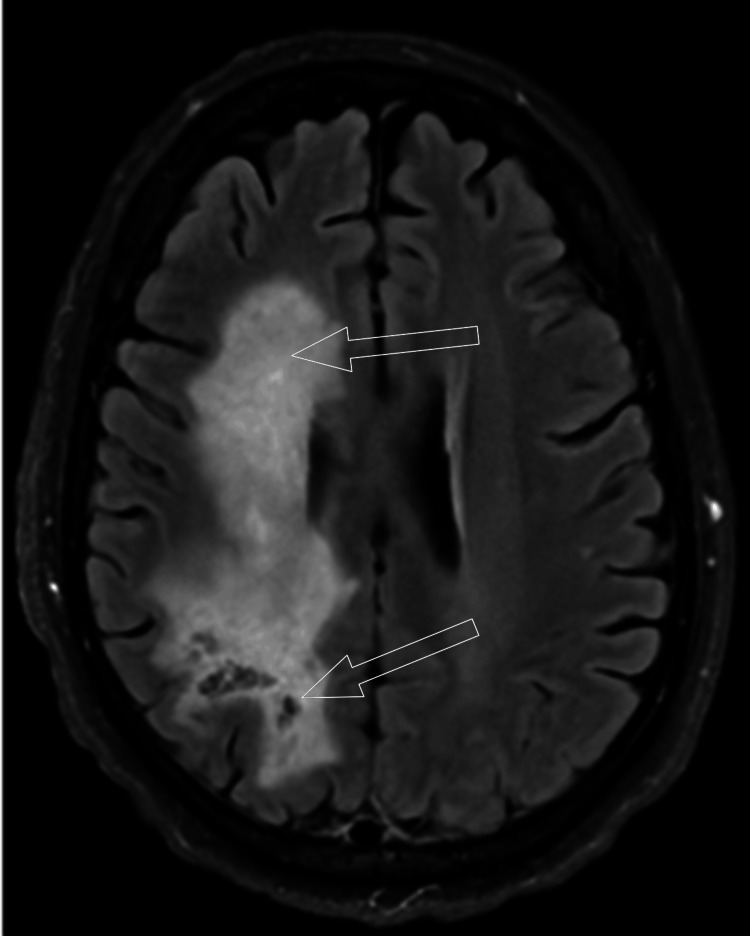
Continued evolving on FLAIR Post-treatment changes on the T2 fluid-attenuated inversion recovery (FLAIR) sequences. The caudal unfilled arrows demonstrate signal loss consistent with gliosis as opposed to the frontal arrow, which shows a persistent, uniform abnormal signal.
